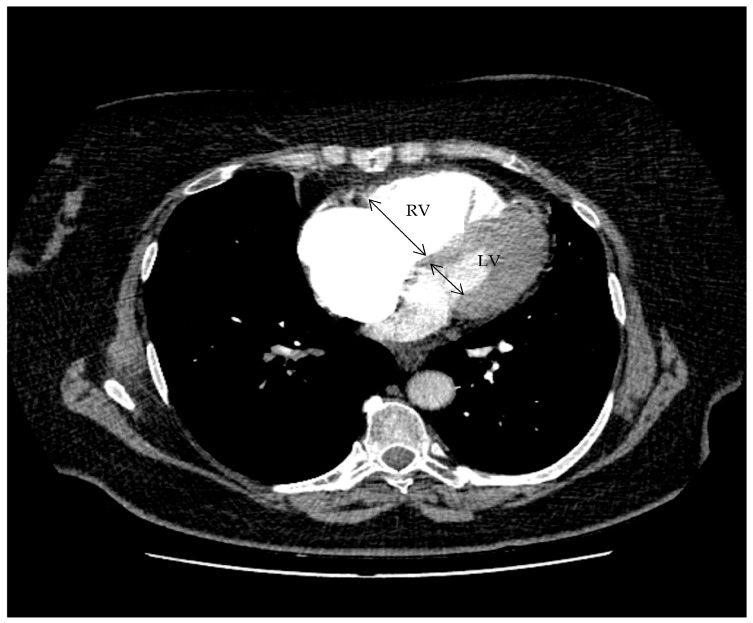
Measurement of the right-to-left ventricular diameter ratio on axial CTPA. Axial contrast-enhanced CT image at the level of the ventricles demonstrating measurement of the maximal transverse diameter of the right ventricle and left ventricle. The RV/LV ratio was calculated as the RV diameter divided by the LV diameter.
